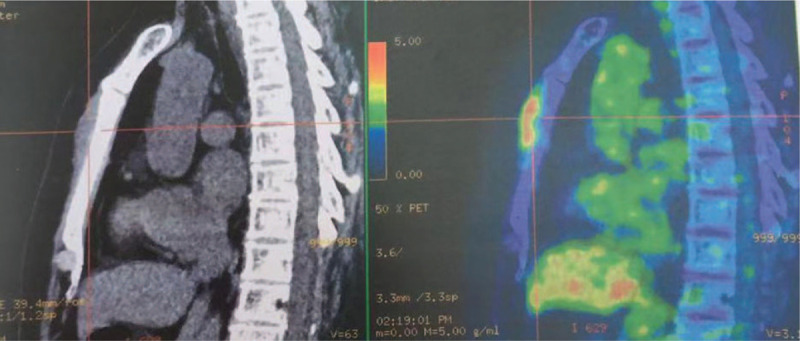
18FDG-PET-CT revealed a mass anterior to the body of the sternum and FDG uptake in the lesion, with a maximal standardize uptake value (SUVmax) of 5.7 on October 28, 2019.
